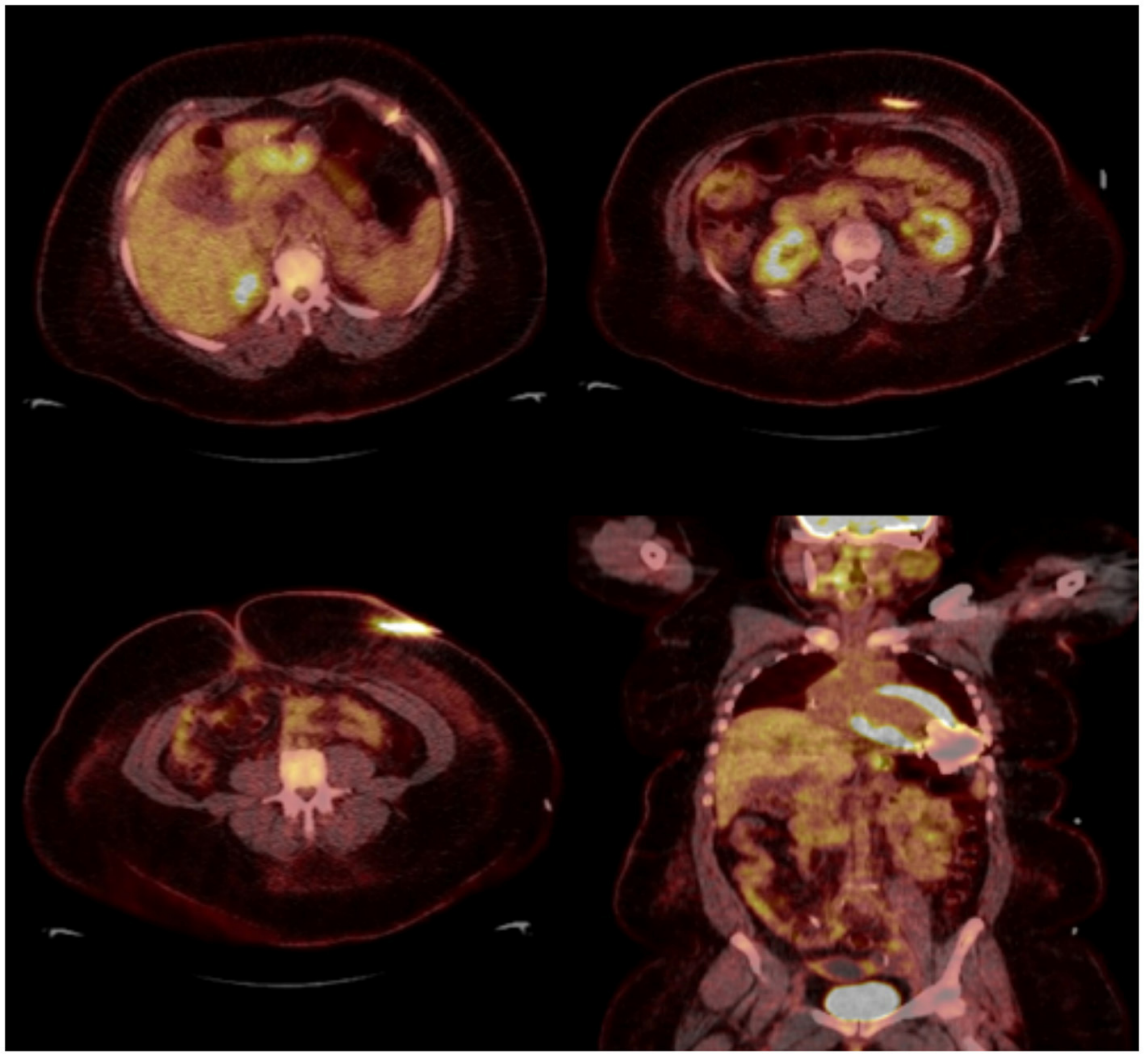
Thirty eight year-old female with end-stage heart failure s/p LVAD (Heart Mate 3) presented with drainage around driveline exit site and abdominal pain. She was treated with oral antibiotics for several weeks but continued to have symptoms. ^18^F-FDG PET-CT was obtained to assess extent of infection and showed increase FDG uptake surrounding LVAD driveline in the anterior abdominal wall without collections. She underwent driveline debridement for DLI and the driveline exit was relocated medially.
